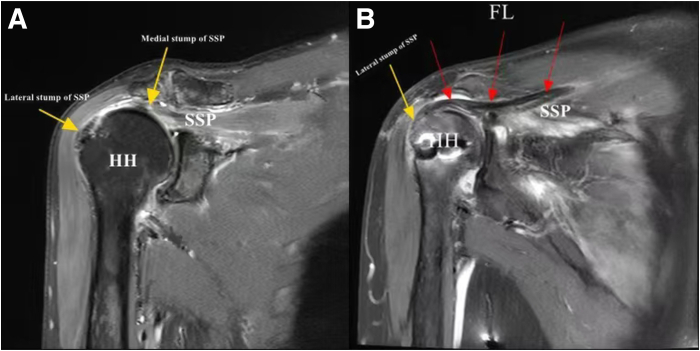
Magnetic resonance imaging findings. (A) Preoperative coronal T2-weighted fat-suppressed image showing musculotendinous junction injury with partial preservation of distal tendon tissue (yellow arrows). (B) Six-month postoperative coronal T2-weighted fat-suppressed image showing successful integration of both the preserved distal tendon (yellow arrow) and graft with the supraspinatus muscle belly (red arrows). (FL, fascia lata; HH, humeral head; SSP, supraspinatus.)
